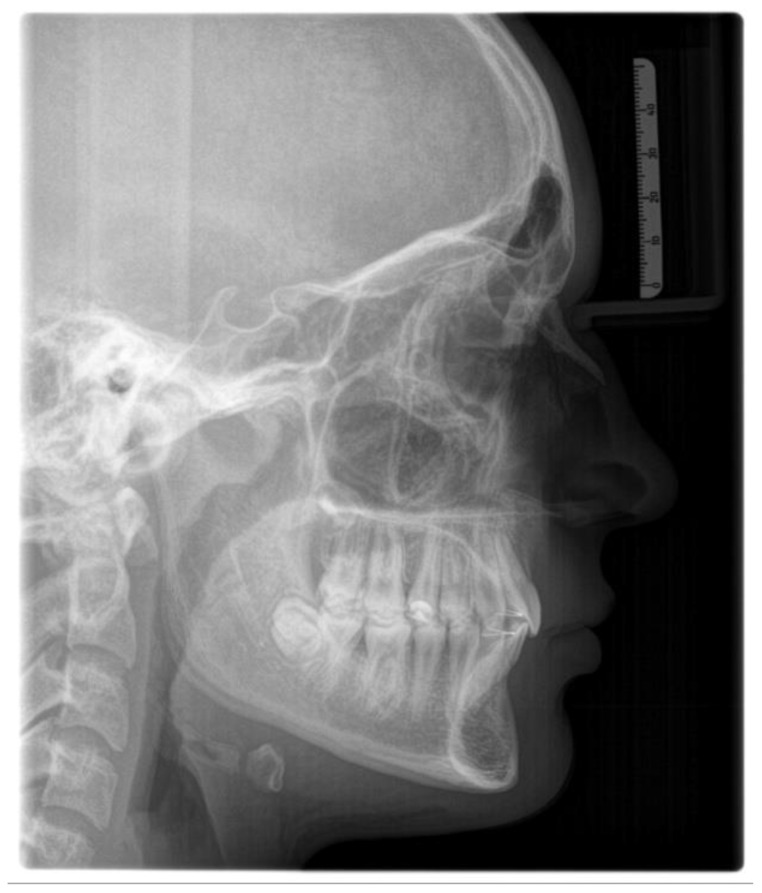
Lateral cephalometric radiograph.
